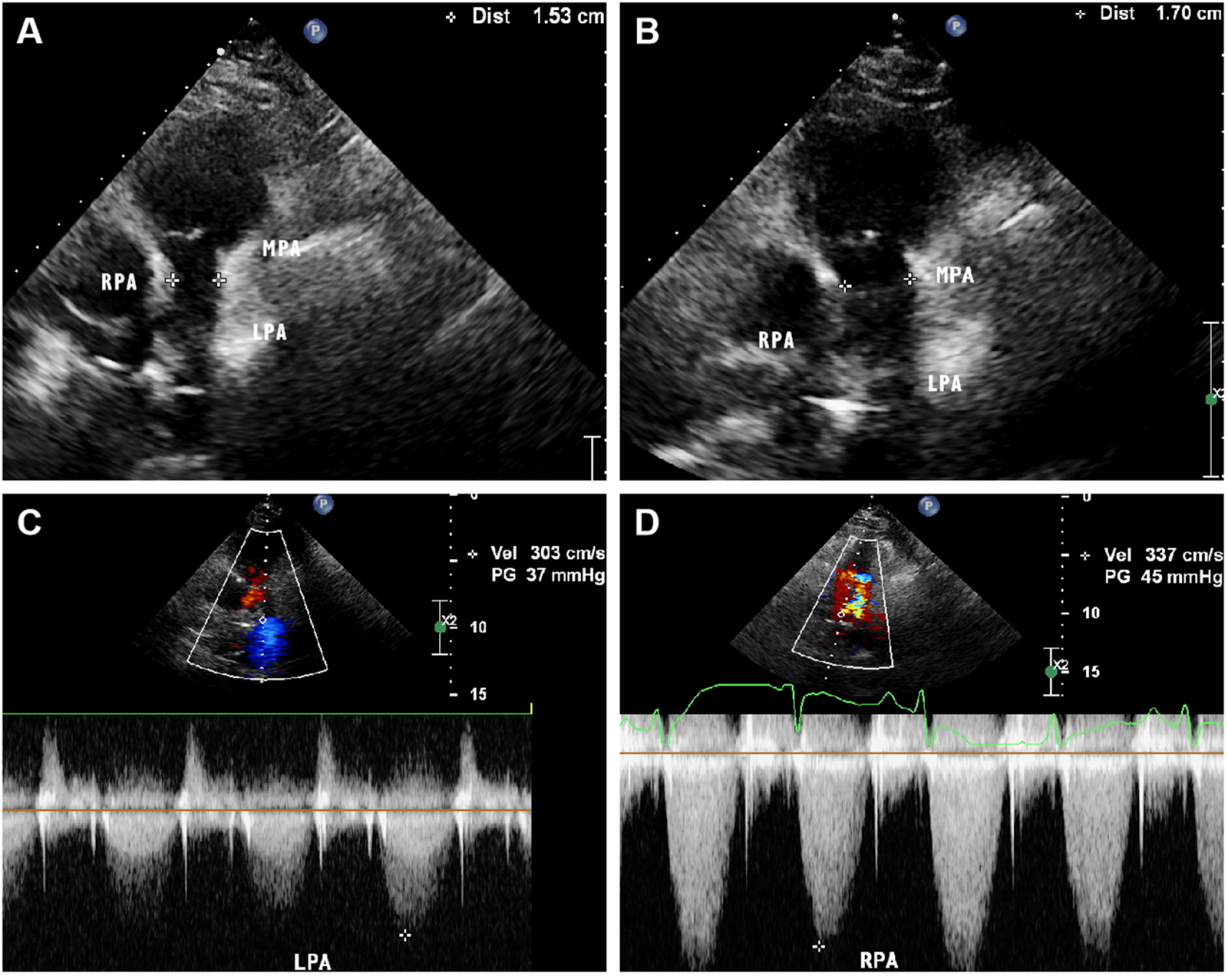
Parasternal pulmonary artery long axis view of transthoracic echocardiography on admission showed narrowing of the main pulmonary artery lumen with an internal diameter of 15 mm. (B) Parasternal pulmonary artery long axis view of transthoracic echocardiography after treatment showed a slight improvement in the narrowing of the main pulmonary artery, with an internal diameter of 17 mm. (C,D) Color Doppler showed that the systolic blood flow velocity of left and right pulmonary artery was about 3.0 m/s and 3.3 m/s, respectively.
